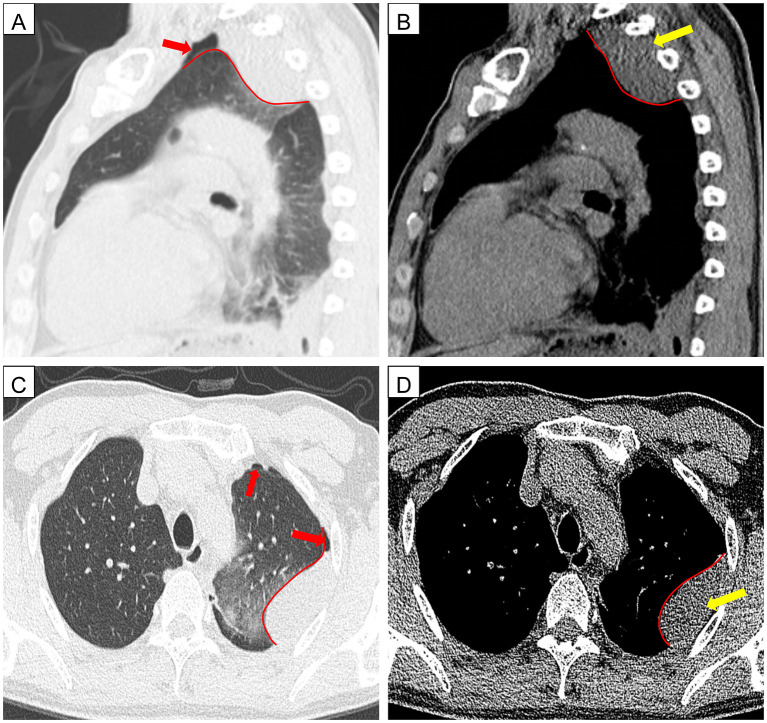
Admission chest CT showed loculated hydropneumothorax, which was further confirmed as pyopneumothorax intraoperatively. (A, B) Sagittal images; (C, D) Axial images. Red solid arrows indicate loculated pneumothorax, yellow arrows indicate pleural effusion, and the red solid line indicates the border of compressive atelectasis of the left lung.
